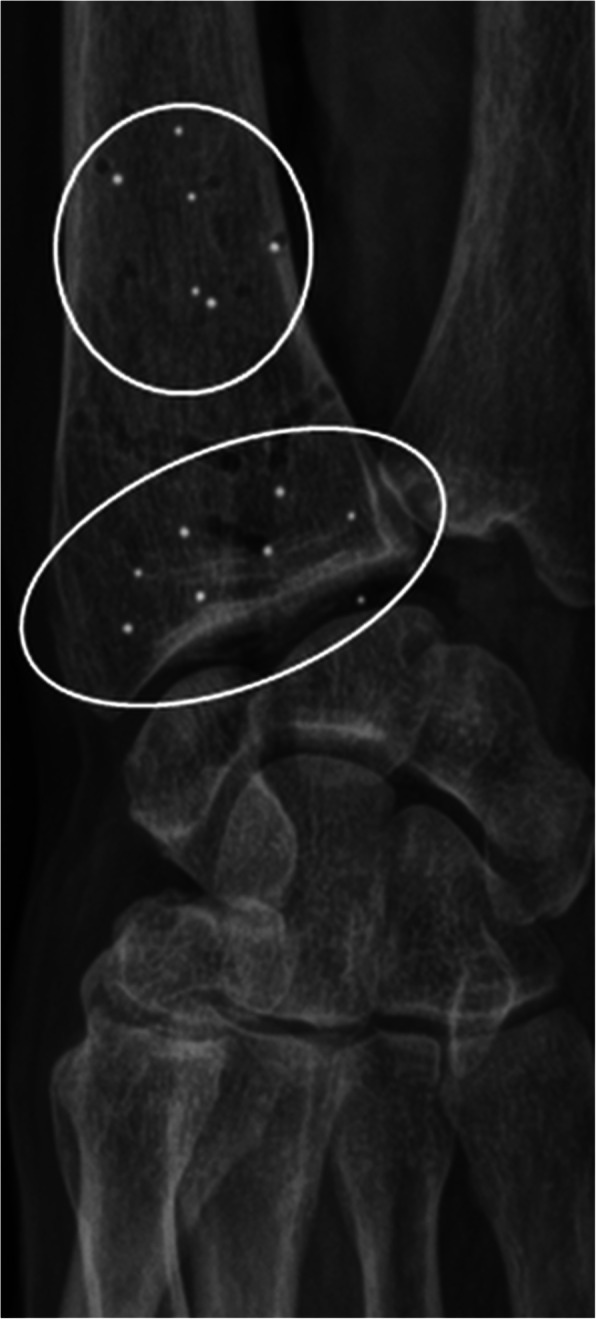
Cross-table posterior-anterior radiograph of a donor arm. Proximal and distal segments (white circles) with tantalum markers
